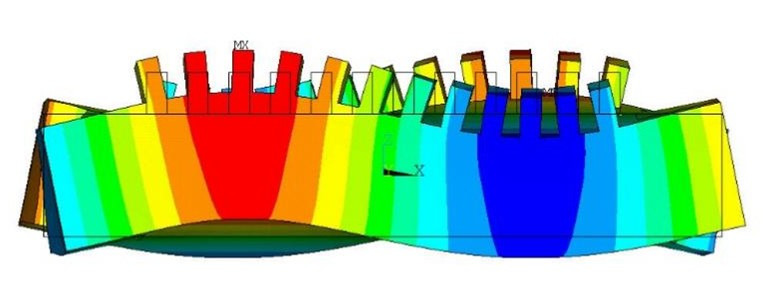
Vibration modes under harmonic response of the bidirectional self-propelled traveling-wave linear ultrasonic motor.
